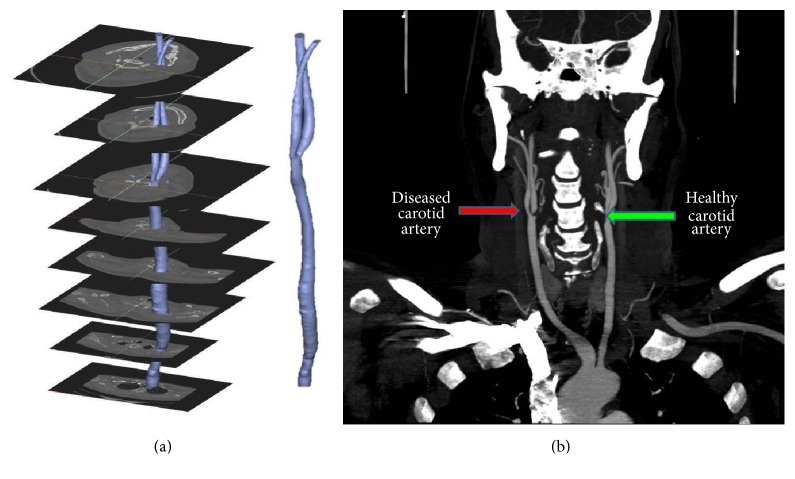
(a) 3D reconstruction of CAs from stack of CT scan slices (images); (b) front view of CT image showing RCA (diseased) and LCA (healthy).
